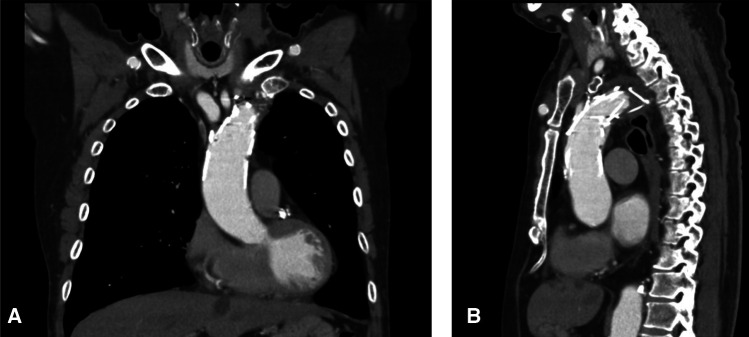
Postoperative CT showed no bird-beak phenomenon both in the coronal (A) and sagittal (B) sections.
